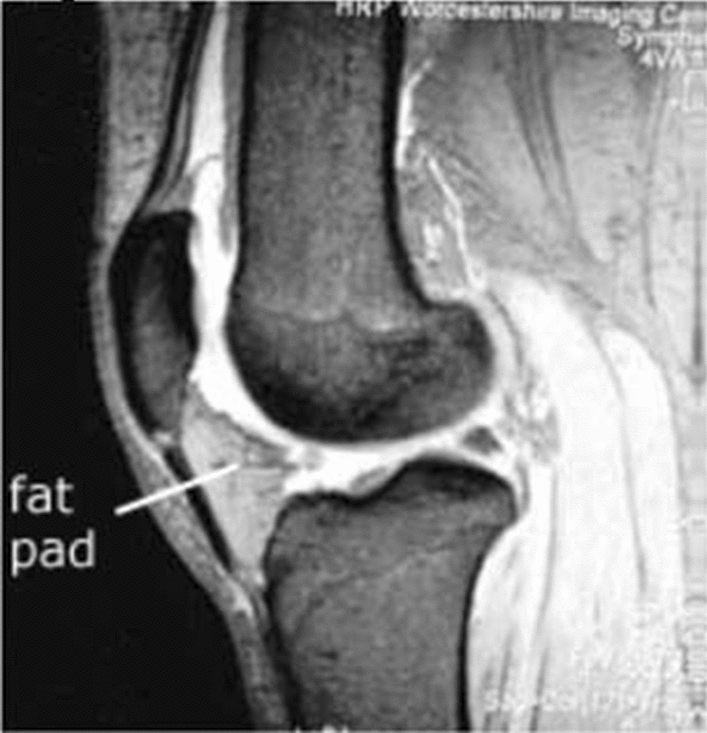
Fat pad in knee joint. 3T sagittal intermediate-weighted (IW) 2D TSE Fat-suppressed MRI sequence
